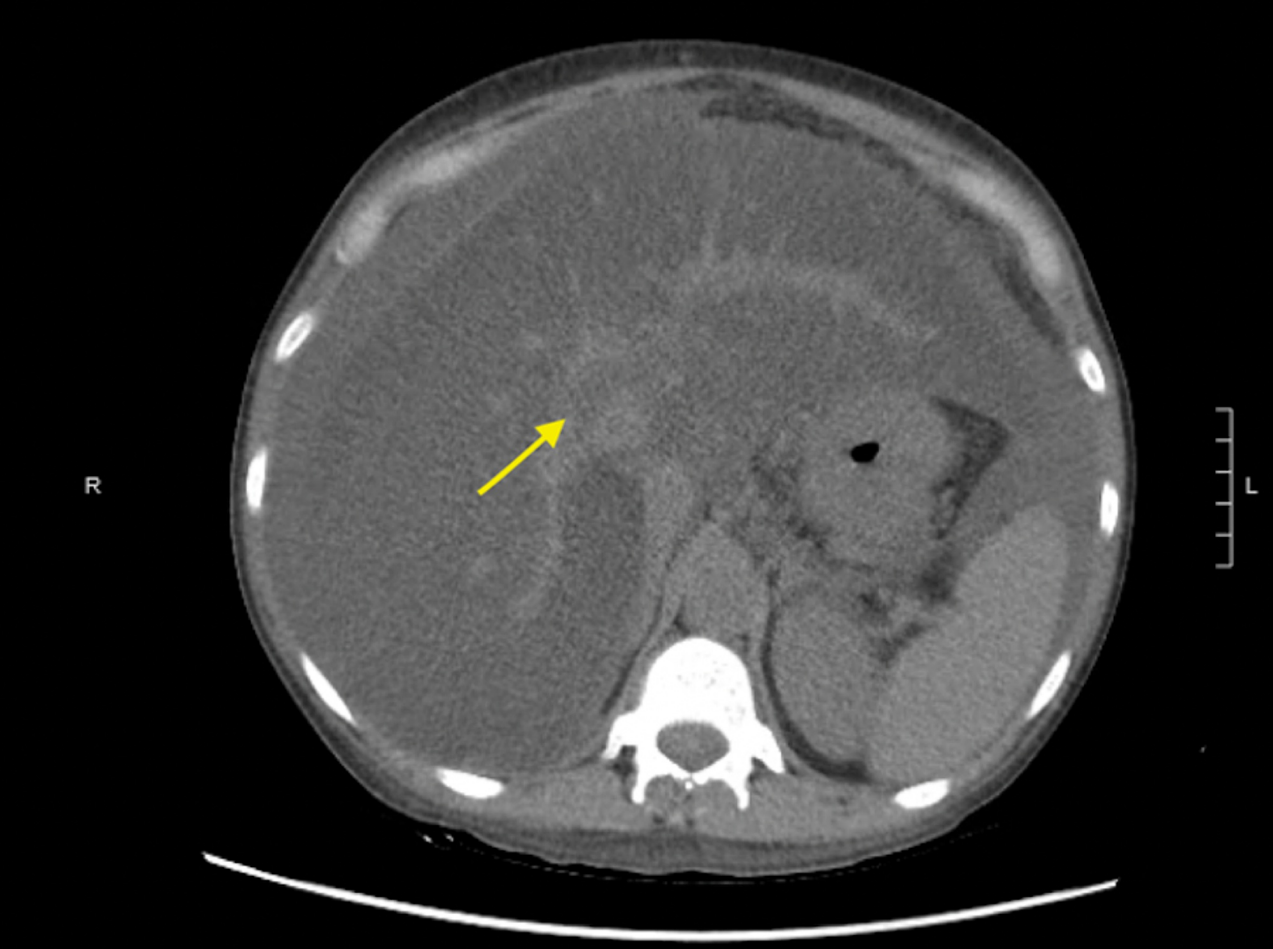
CT of the abdomen without contrast displaying portal vein thrombosis (arrow) CT: computed tomography
